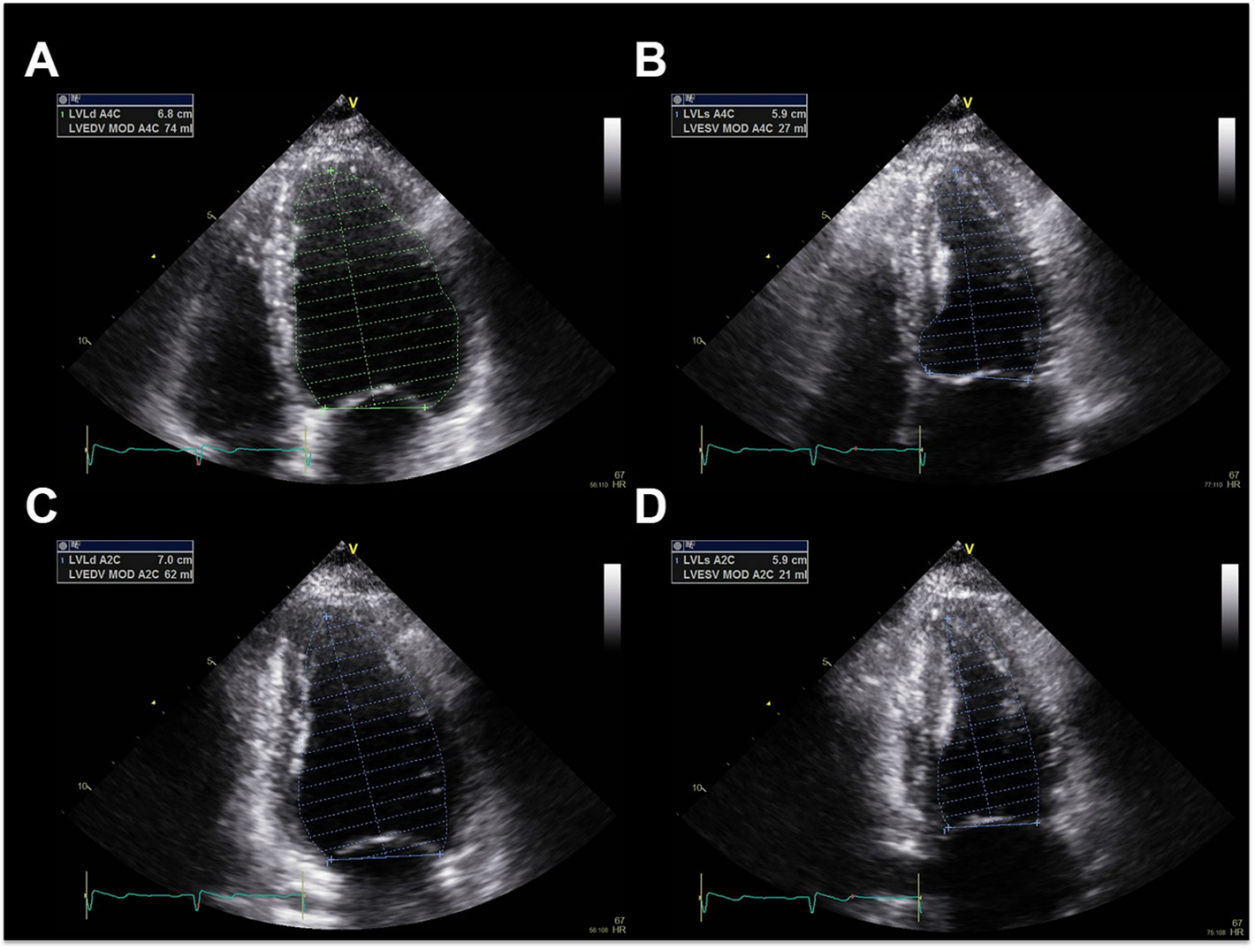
Two-dimensional calculations for volume calculations using biplane methods of disks (modified Simpson’s method) in apical 4 chamber (A and B) and apical 2 chamber views (C and D). Ejection fraction can be measured from the division of the stroke volume (subtraction of end-systolic volume from end-diastolic volume) by end-diastolic volume.
